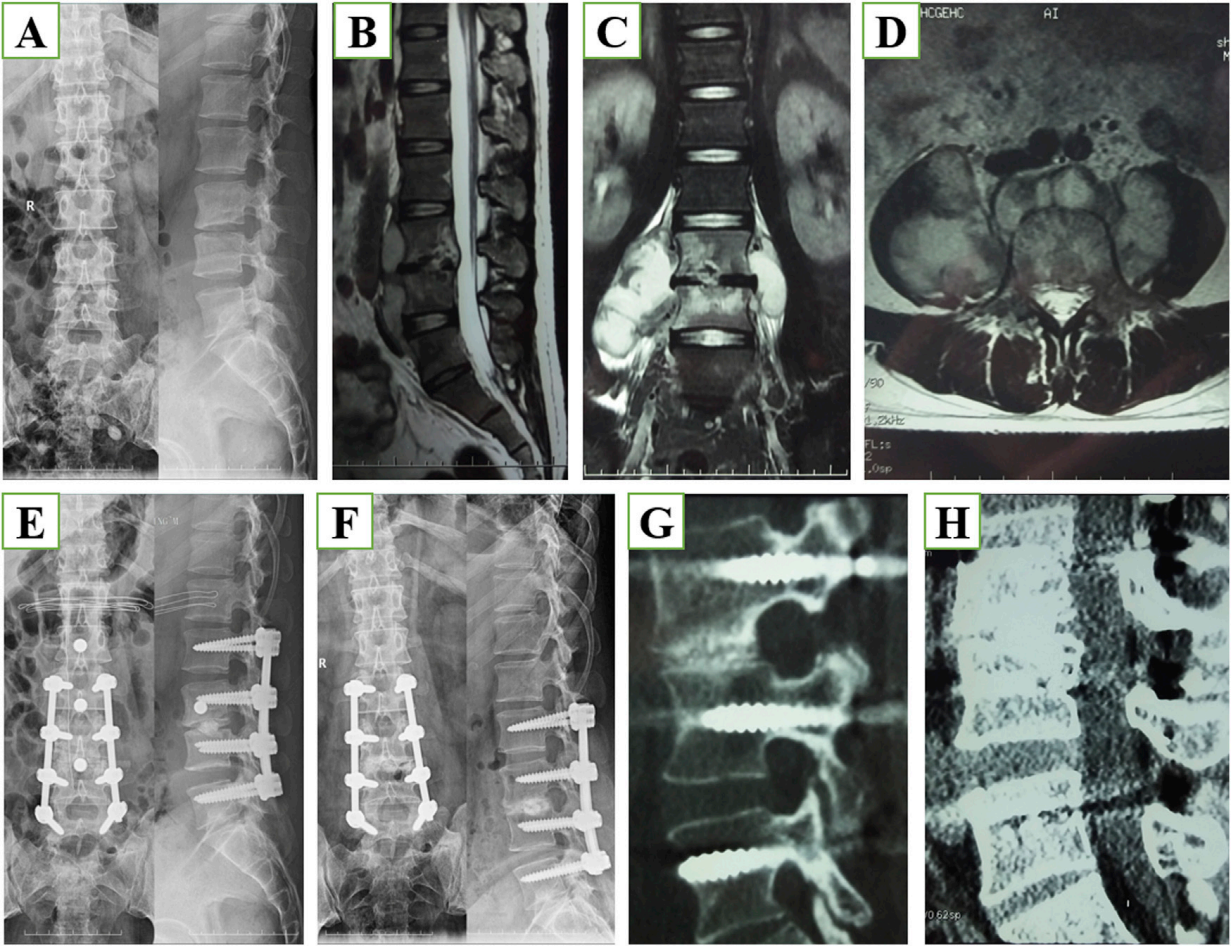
(A) Anterior-posterior and lateral x-ray images before surgery. (B) Magnetic resonance imaging (MRI), sagittal section, of the lumbar spine before surgery. (C) Lumbar MRI coronal section before surgery. (D) Lumbar MRI axial section before surgery. (E) X-ray images immediately after surgery. (F) X-ray images 10 months after surgery. (G) Computed tomography (CT) using the bone window 10 months after surgery. (H) CT using soft tissue window 10 months after surgery.
